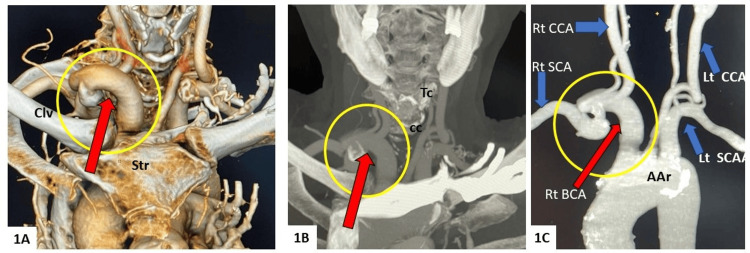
3D volume rendered image (A) and MIP reconstruction from CT angiography study (B and C) showing high riding tortuous right brachiocephalic artery (encircled, red arrow), rising well above suprastrernal notch. C) Bone subtracted maximum intensity projection (MIP) image Clv: clavicle, Str: sternum, Tc: thyroid cartilage, cc: cricoid cartilage, BCA: brachiocephalic artery. SCA: subclavian artery, CCA: common carotid artery, AAr: aortic arch
